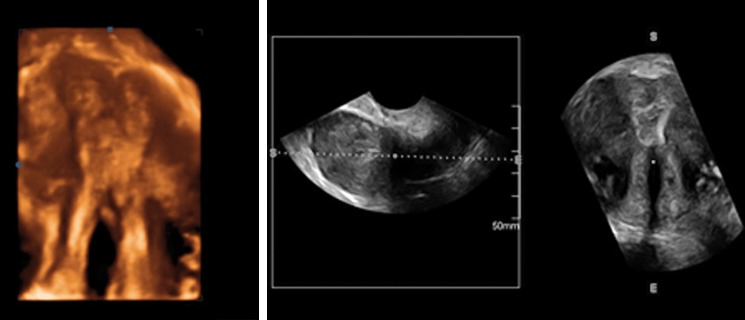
3-D ultrasound image after metroplasty.
